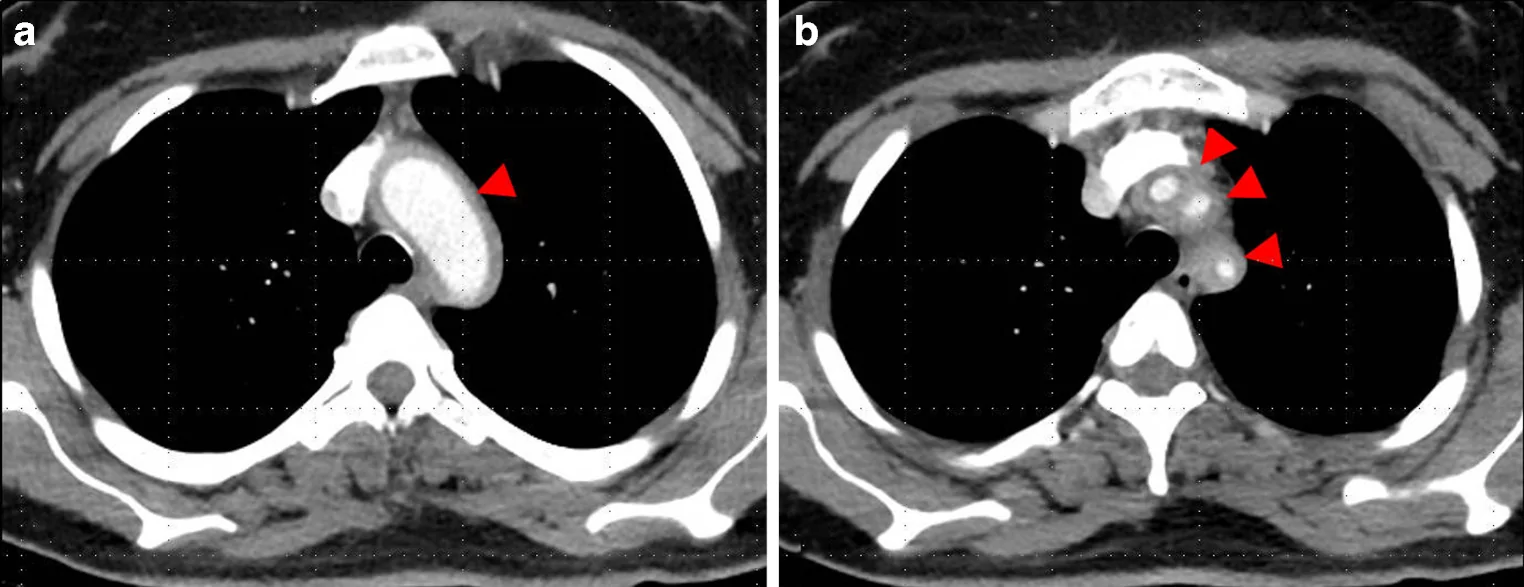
Giant cell arteritis (Takayasu arteritis) in a 34-year old woman suffering from an acute left hemispheric syndrome. a, b CT angiography showing thickened vessel walls of the aortic arch (a arrowhead) and the proximal supraaortal branches (b arrowhead)
